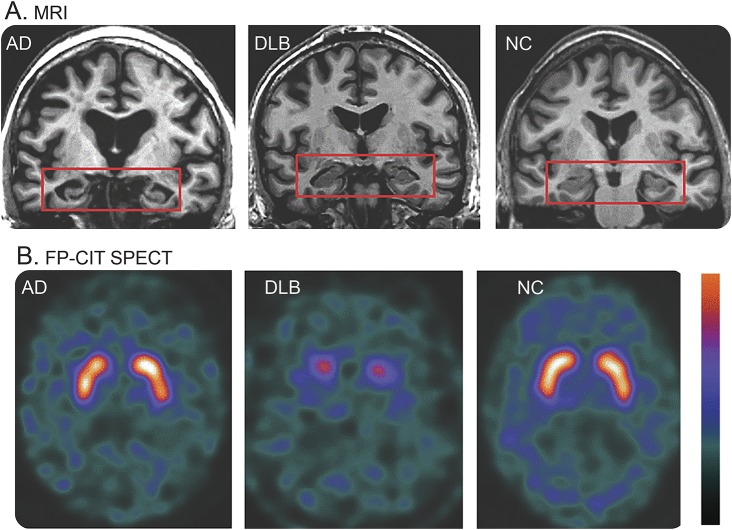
Coronal T1-weighted MRI and ^123^iodine FP-CIT SPECT images in Alzheimer disease (AD), dementia with Lewy bodies (DLB), and normal controls (NC) (A) On the MRI, note the relative preservation of medial temporal lobe volume (rectangles) in DLB, which is similar to NC, whereas atrophy is obvious in AD. (B) On the FP-CIT SPECT images, note the minimal uptake in DLB, which is restricted to the caudate (period or full-stop appearance) compared to the robust uptake in the caudate and putamen in AD and NC (comma appearance). Reproduced with permission from Dr. Val Lowe, Mayo Clinic, Rochester, MN.
